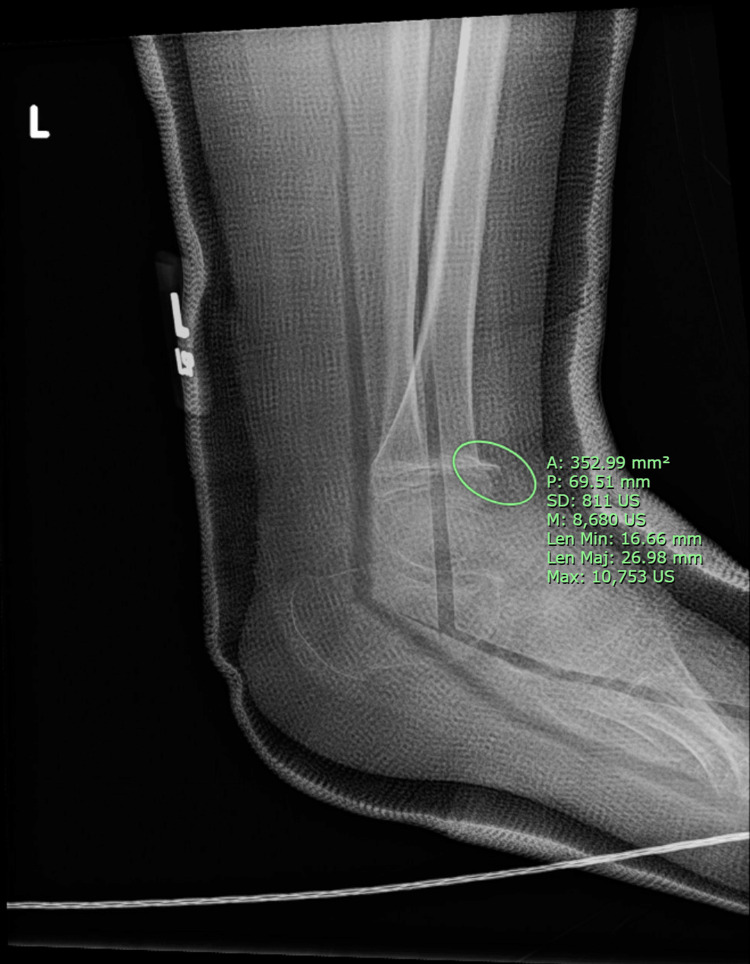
Left ankle X-ray showing a nondisplaced buckle fracture of the anterior metaphysis of the distal tibia
